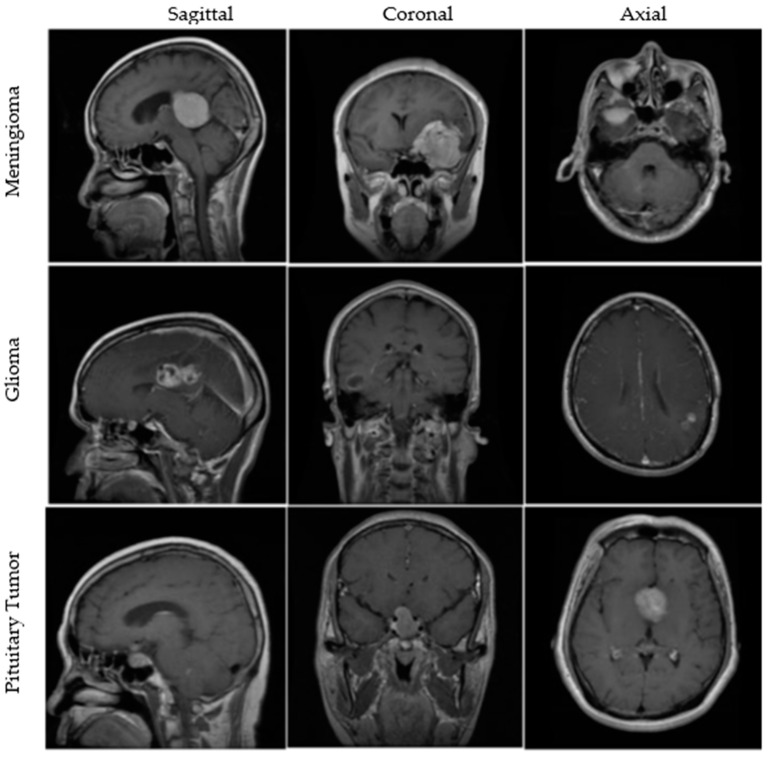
MRI images from the figshare dataset are shown in sagittal, coronal, and axial cut planes for meningiomas, gliomas, and pituitary tumors.
